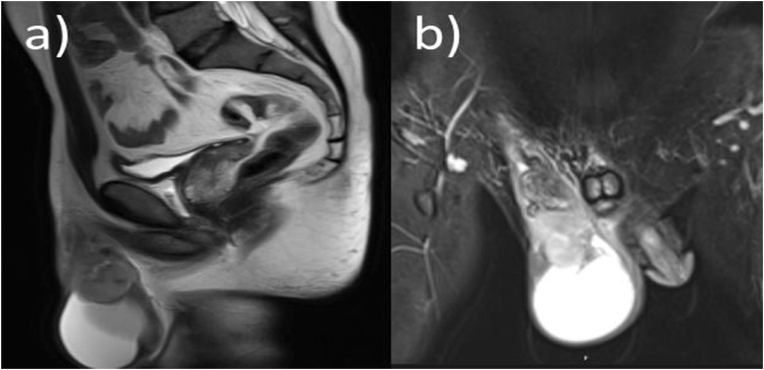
Pelvic magnetic resonance imaging demonstrating a well-defined, heterogeneous solid mass arising from the spermatic cord in the paratesticular region. The lesion shows predominantly intermediate signal intensity on T1-weighted images and heterogeneous high signal intensity on T2-weighted images, with displacement of adjacent scrotal structures and no evidence of direct testicular invasion.
